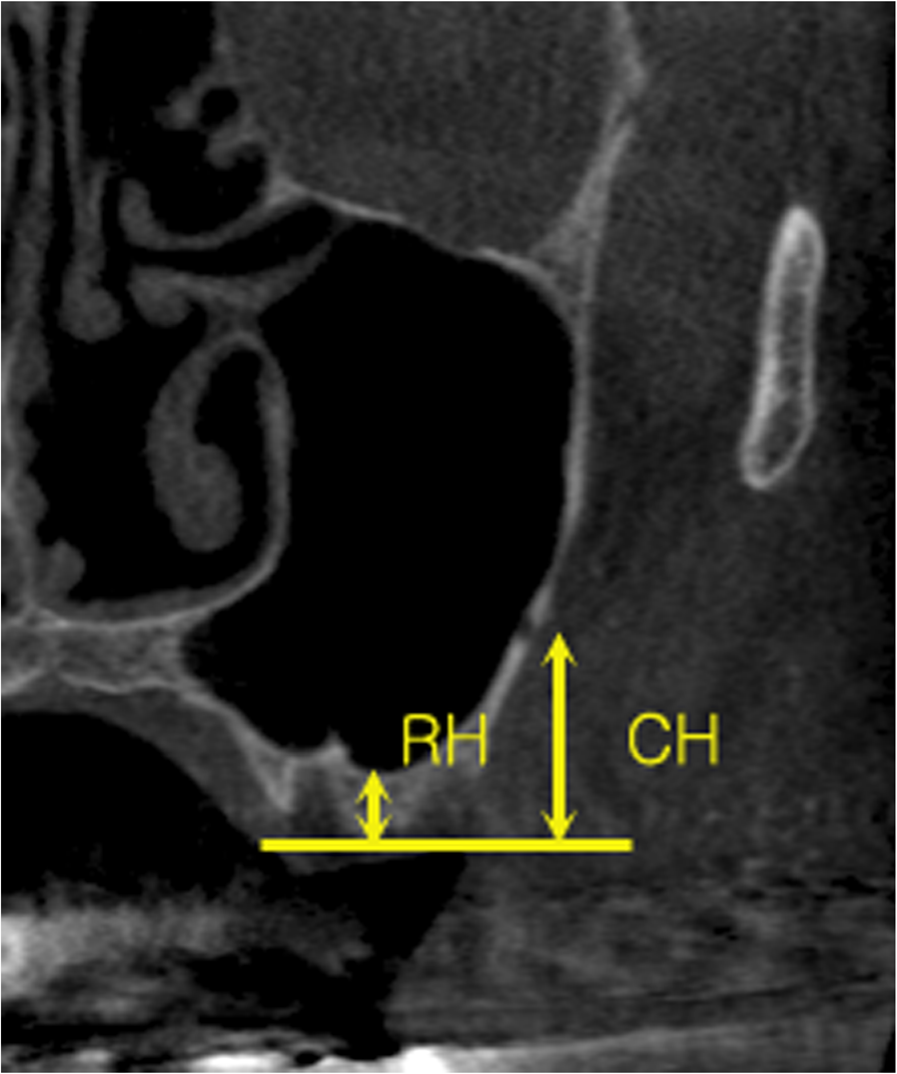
The vertical position of the bony canals according to the tooth region on para-axial sinus CT scan. *CH* (canal height), distance between alveolar crest and inferior border of the canal; *RH* (ridge height), distance between alveolar crest and sinus floor; *CHS* (canal height from the sinus); *CH*-*RH*
